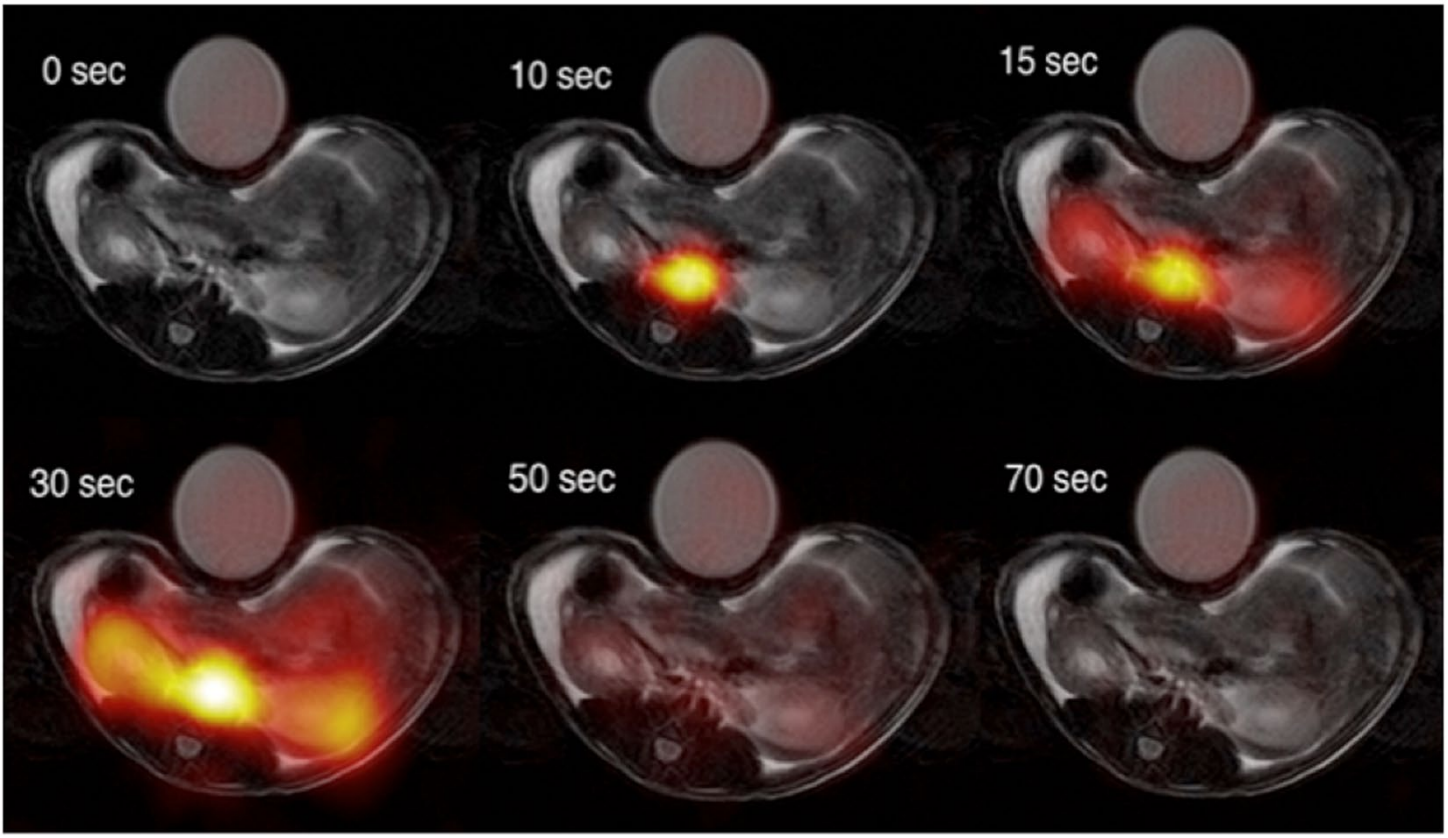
Examples of [1-^13^C]-acetate uptake in the aorta and kidneys over time. Hyperpolarized [1-^13^C]-acetate signal overlaid ^1^H-anatomical MR images of an axial slice, showing two kidneys and the presence of a signal in the aorta and following the kidneys. MR; magnetic resonance.
